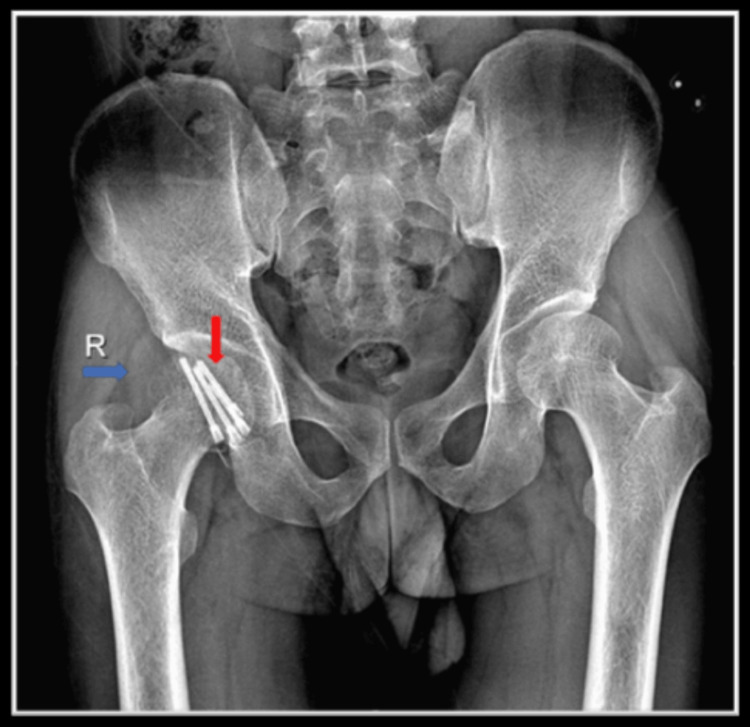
Postoperative plain radiograph of pelvis anteroposterior view showing Herbert screws (shown by red arrow) and the elevation of fat plane (shown by blue arrow)
